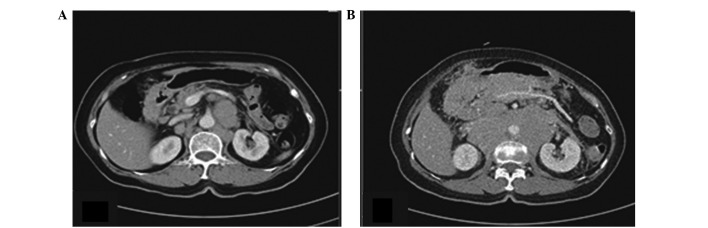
Abdominal computed tomography scan providing sequencing images at (A) diagnosis and (B) following one month of treatment. (A) MRI scan shwoing mass lesions in the wall of the gastric antrum and duodenal bulb, as well as enlarged lymph nodes in the abdominal and retroperitoneal regions. (B) Following treatment the abdominal mass enlarged with extensive lymph node metastasis.
